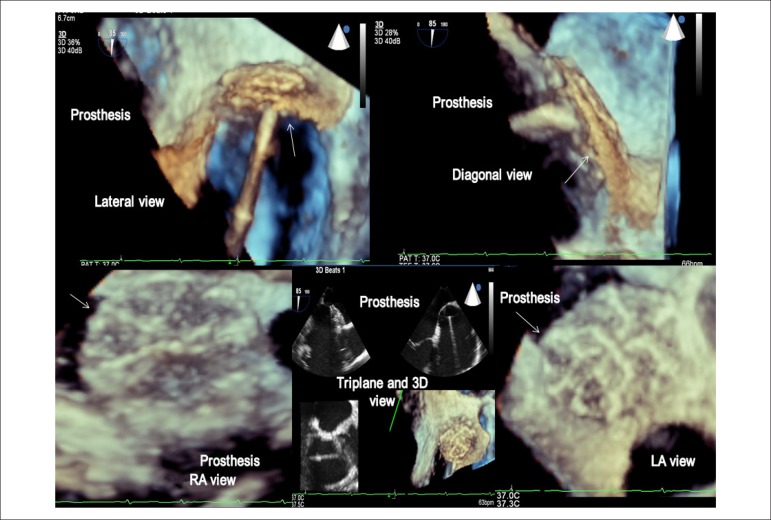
Three-dimensional transesophageal echocardiography image in multiple planes
